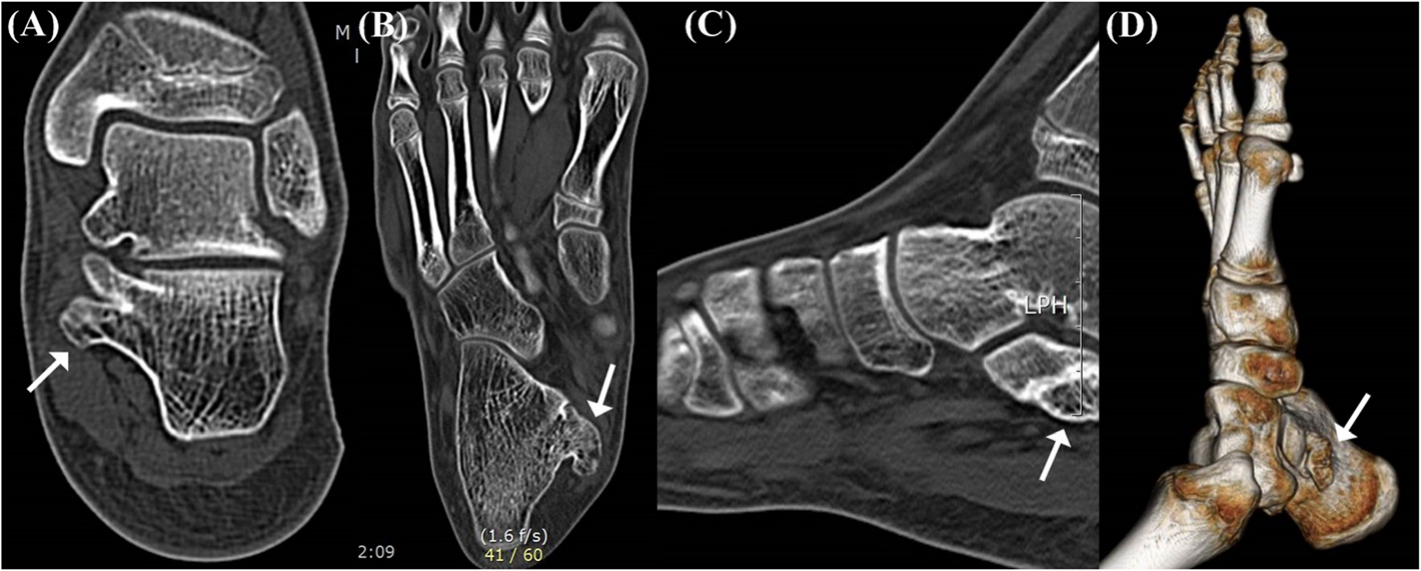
Preoperative CT scans of the left foot. Coronal (a), axial (b), sagittal (c), and three dimensional reconstructed (d) views show 2.5 × 1 cm sized bony protuberance below the sustentaculum tali, demonstrating an apparent corticomedullary continuity with the underlying bone (arrows). Neither talocalcaneal coalition nor fracture within the bony protuberance is detected
